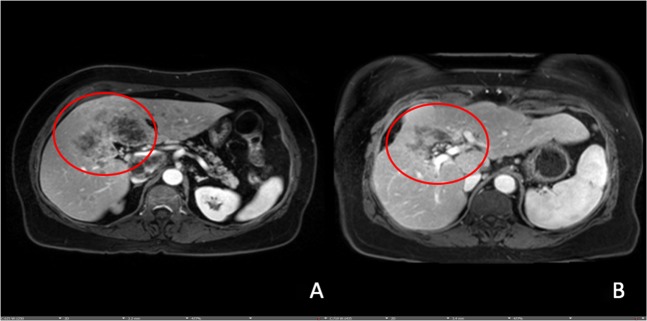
MRI pre- and post-treatment with decreased size and enhancement of lesions in liver
